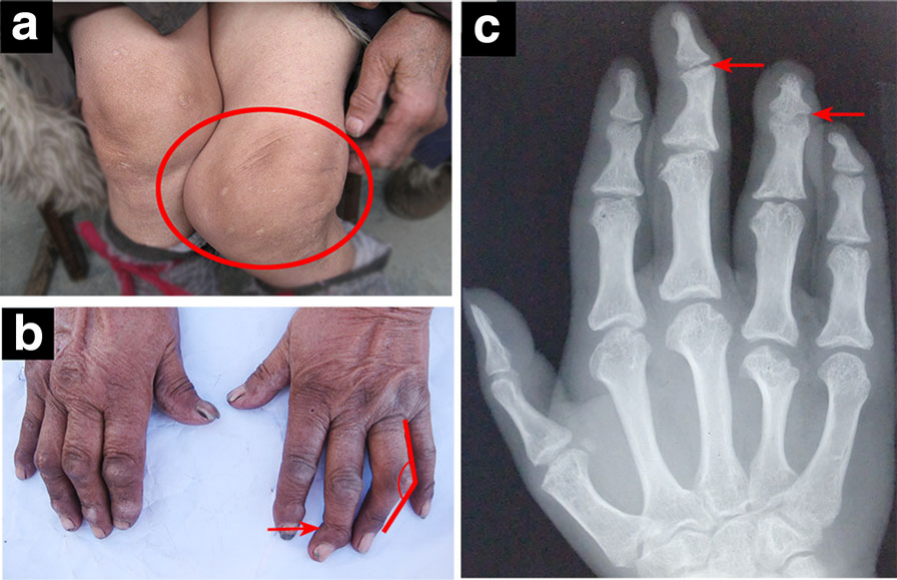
Clinical features of patients with Kashin-Beck disease (KBD). a and b A patient with KBD shows obvious flexion and extension in the knees (*circled area*) and inter-phalangeal joints (*arrow and angle labeled area*), with deformity and limited mobility. c The x-ray radiographs of a KBD patient with irregular, enlarged hand inter-phalangeal joints
